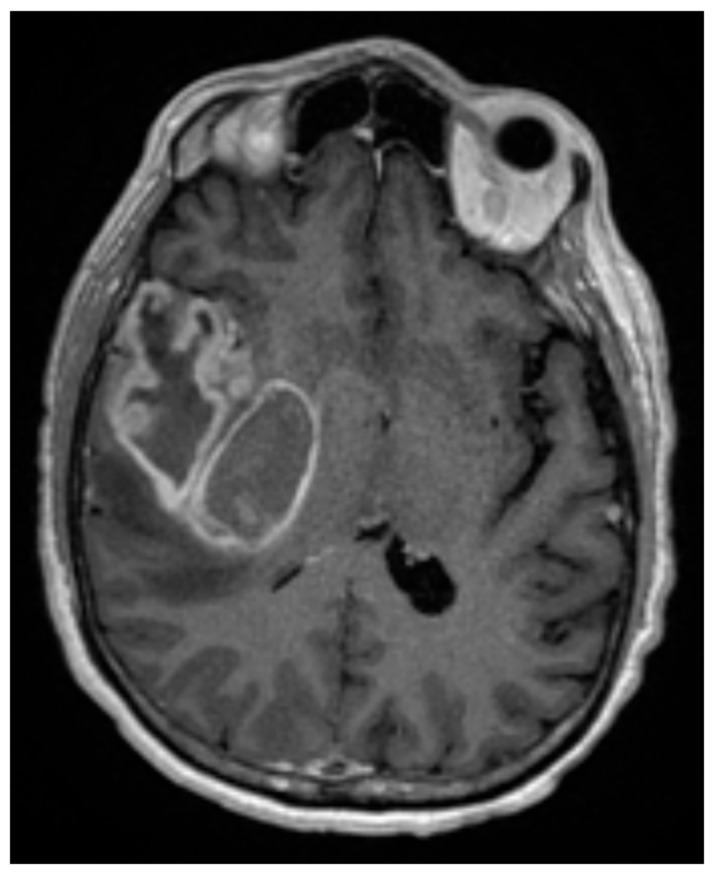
T1 post-contrast axial MRI of a 60-year-old man who presented with a 3-month history of progressively worsening headaches and memory loss. The MRI was consistent with a large contrast-enhancing mass in the right temporal lobe. He underwent a gross total resection, which demonstrated a 1p19q intact, IDH-wildtype, WHO grade 4 glioma, MGMT promoter unmethylated. Surgery was followed by concurrent external beam radiation therapy (60 Gy in 30 fractions) with concurrent TMZ, followed by adjuvant TMZ and TTF. He succumbed to his disease at 13 months following diagnosis.
